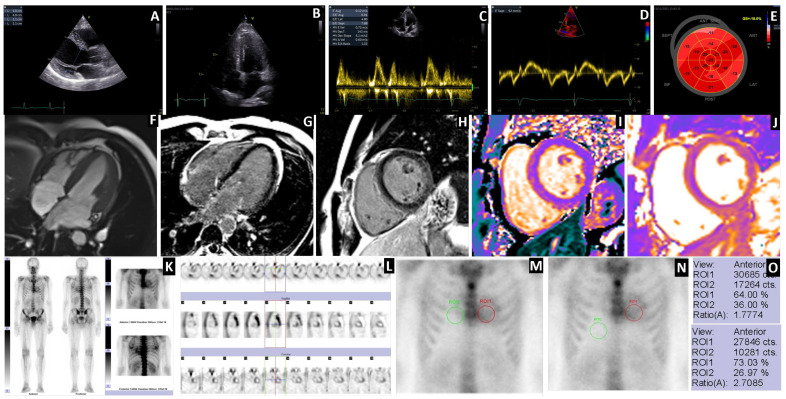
Youngest patient with early cardiac involvement with G2 score. Transthoracic echocardiography (A–E). Parasternal long axis diastolic frame (A) and apical 4-chamber systolic frame (B) of the youngest patient presented with normal cavity sizes and function. Pulsed wave Doppler with the sample volume located at the tip of the mitral leaflets (C) and Tissue Doppler echocardiography with the sample volume located at the base of the septal mitral annulus (D), revealing normal diastolic and longitudinal function. Automated function imaging derived from the speckle tracking echocardiography (E), revealing a normal GLS with a normal pattern of the Bull’s eye view. Contrast-enhanced cardiac magnetic resonance (F–J). Cine (balanced SSFP) 4-chamber sequences (F) revealed the same findings as the transthoracic echocardiography. Late gadolinium enhancement sequences in 4 chambers and basal short axis show only minor, subendocardial LGE, more prominent on the right side of the interventricular septum with normal gadolinium kinetics (G,H). Noncontrast T1 mapping acquired on a 1.5 T scanner revealed a less elevated native T1 of 1126 ms corresponding to a less severe extracellular expansion (ECV 38%) (I). A normal native T2 (44 ms), demonstrating no evidence of myocardial edema (J). Bisphosphonate scintigraphy (K–N). Planar Whole-Body acquisition in the anterior and posterior incidence from the vertex to the soles. Static acquisition at the chest level in the anterior and posterior views showing diffusely increased uptake at the heart level, of the same intensity as the costal one, with a Perugini visual uptake score of G2 (K). Acquisition of SPECT at the chest level, with the delimitation of the LV walls and free wall of the RV, after the reconstruction and display of the transverse, sagittal, and coronal slices (L). Selection of ROIs from the LV level and the contralateral region where the RV overlaps (M). Selection of ROIs at the LV level and liver regions, without including the RV (N). The difference in the semiquantitative score of the H/CL (*n* = 1.77) and H/L (*n* = 2.70) ratios is due to the overlap of the RV. (O) ECV = extracellular volume; GLS = global longitudinal strain; LGE = late gadolinium enhancement; LV = left ventricle; ROI = region of interest; RV = right ventricle; SPECT = single-photon emission computer tomography; SSFP=steady-state free precession.
